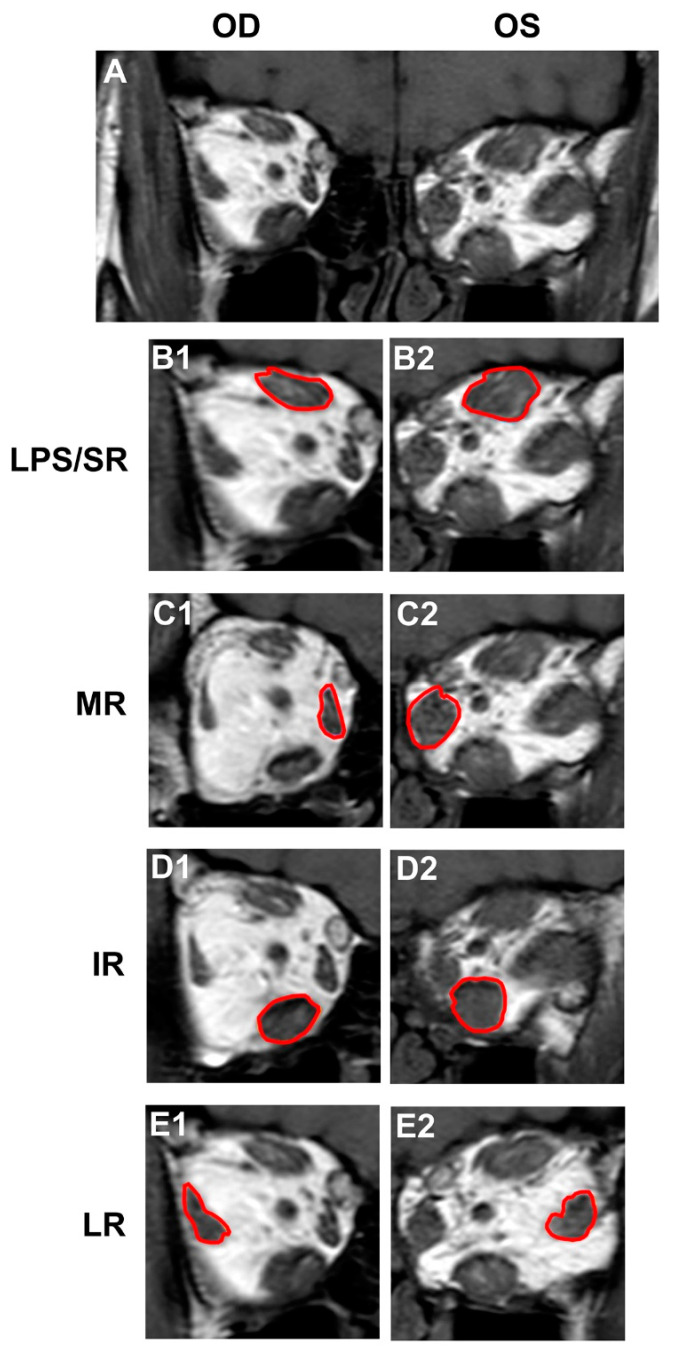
The measurement of the largest cross-sectional area of the extraocular muscle on T1-weighted MR images. (A). Representative T1W orbital MR image. (B1,B2). Segmentation of the largest LPS/SR image. (C1,C2). Segmentation of the largest medial rectus (MR) image. (D1,D2). Segmentation of the largest lateral rectus (LR) image. (E1,E2). Segmentation of the largest inferior rectus (IR) image. Red line represents segmentation of extraocular muscles. LPS/SR, levator palpebrae superioris–superior rectus complex.
